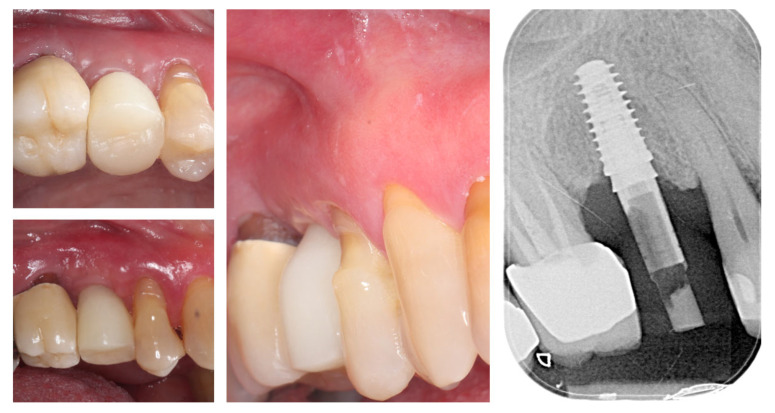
Clinical and radiographic evaluation at 4 months with provisional crown (7 months post-op).
